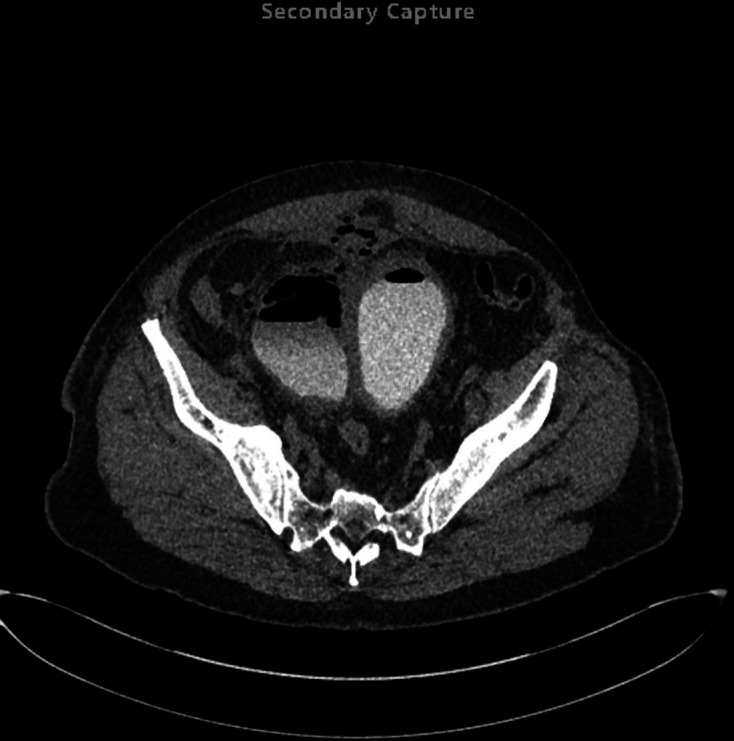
Second axial delayed phase CT (Day 8 of admission), on soft tissue window
